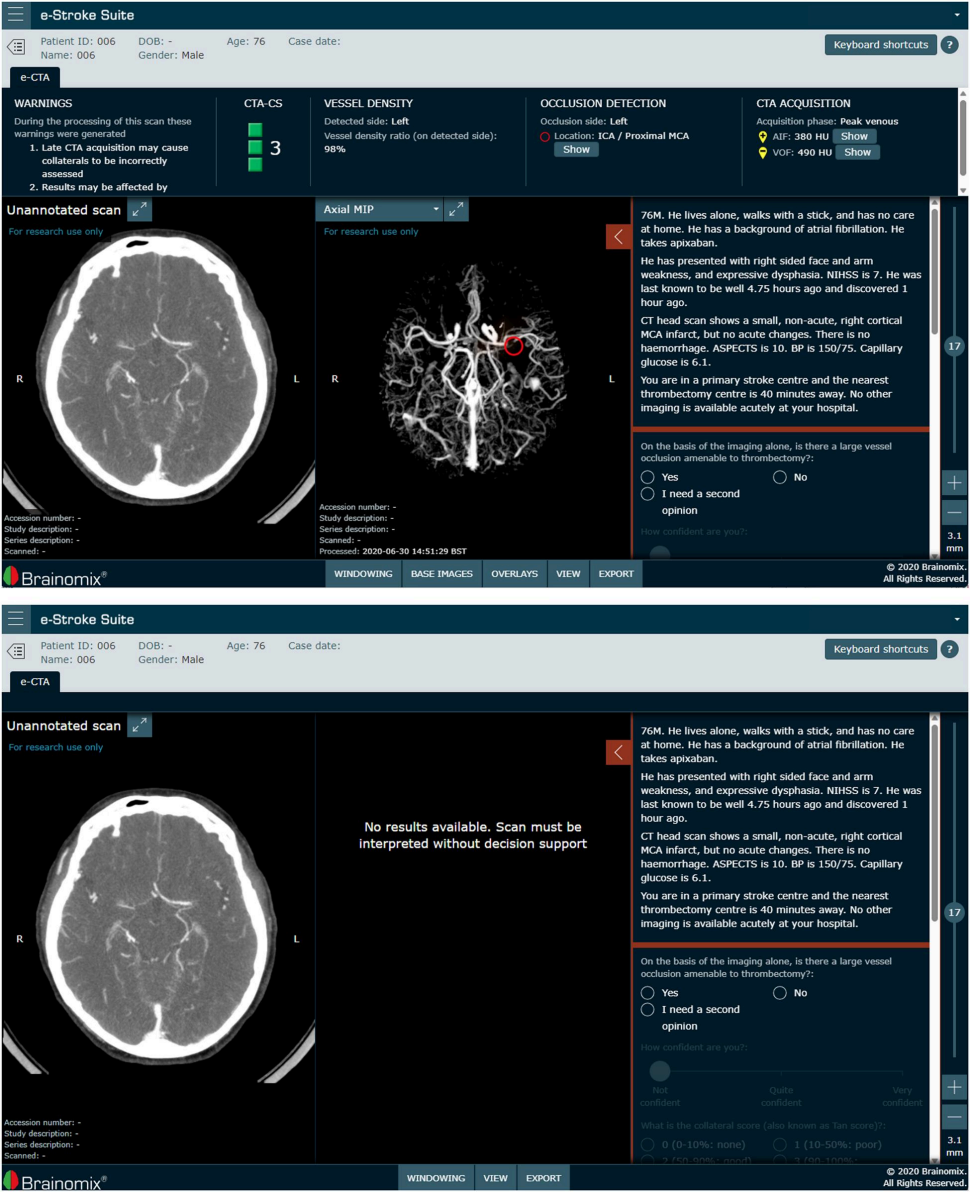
Screenshot examples of the study viewing platform with (top) and without (bottom) decision support from e-CTA.
